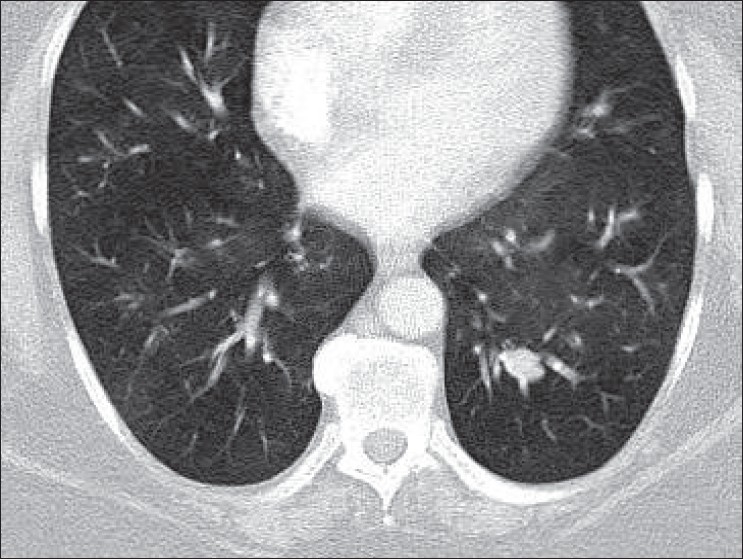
CT chest demonstrating left lower lobe nodule
